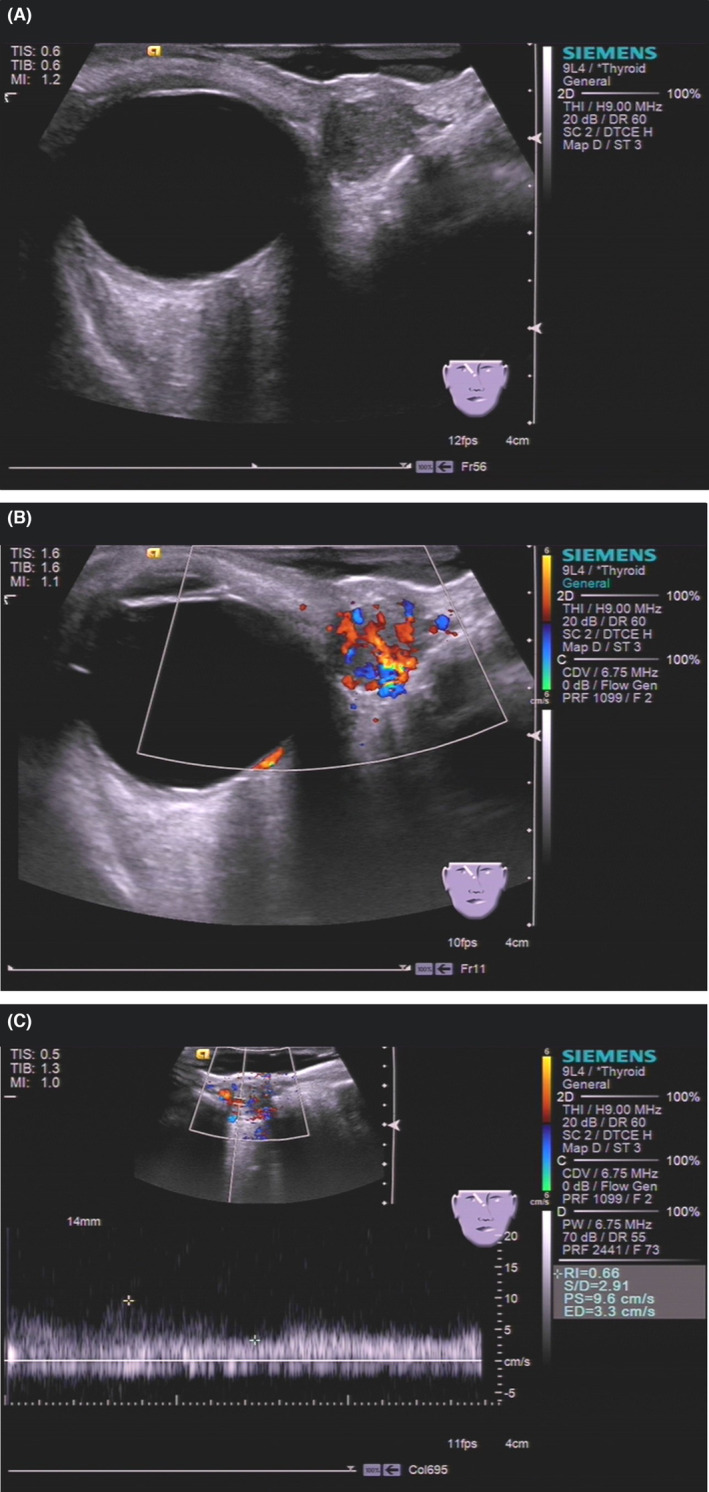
Ultrasonography of superficial angiomyxoma. (A) Grayscale ultrasound showed that the tumor was oval, defined, hypoechoic, homogeneous, and 12 mm × 10 mm in size. (B) Color Doppler flow imaging showed that multiple small and main vessels were visualized inside the tumor. (C) Pulsed‐wave Doppler showed the systolic blood flow velocity was 9.6 cm/s with a high resistance index.
